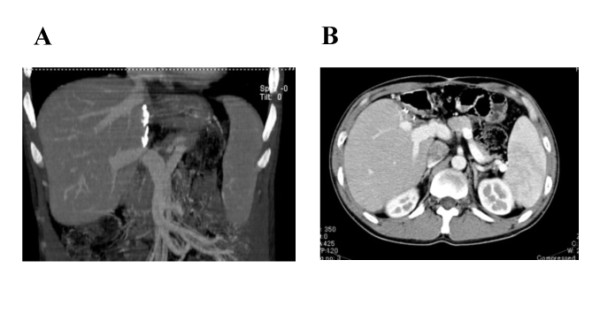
Multi-slice CT imaging one year after the transplantation. The sagittal (A) and enhanced images (B) show that the congestion of the liver completely disappeared with normal flow inside the vessels.
